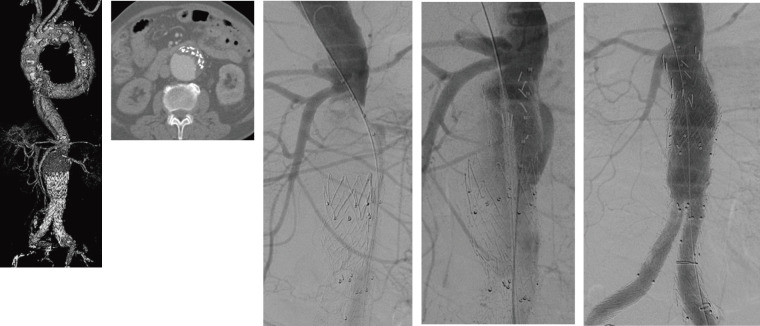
CT showed stent graft compression due to retrograde type B aortic dissection, and additional stenting was performed. CT: computed tomography
